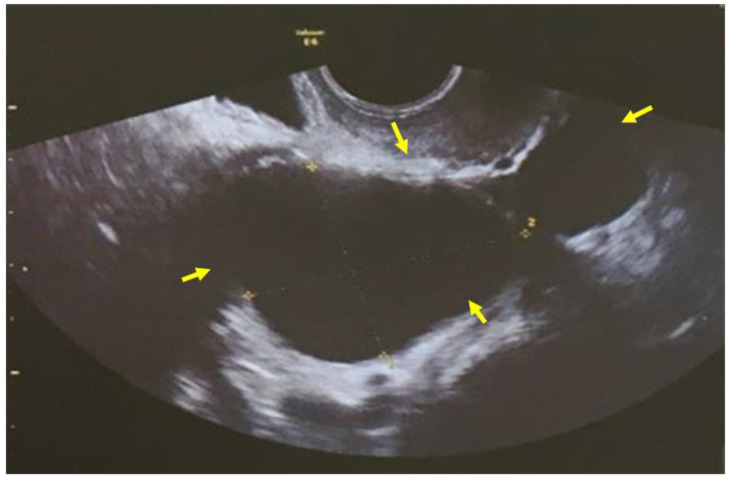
Transvaginal ultrasound imaging: the allantoid form cystic mass at the anatomical position of the right adnexa (yellow arrows) corresponding to primary fallopian tube carcinoma was misdiagnosed as a two-chambered cystic lesion of the ovary (our case).
